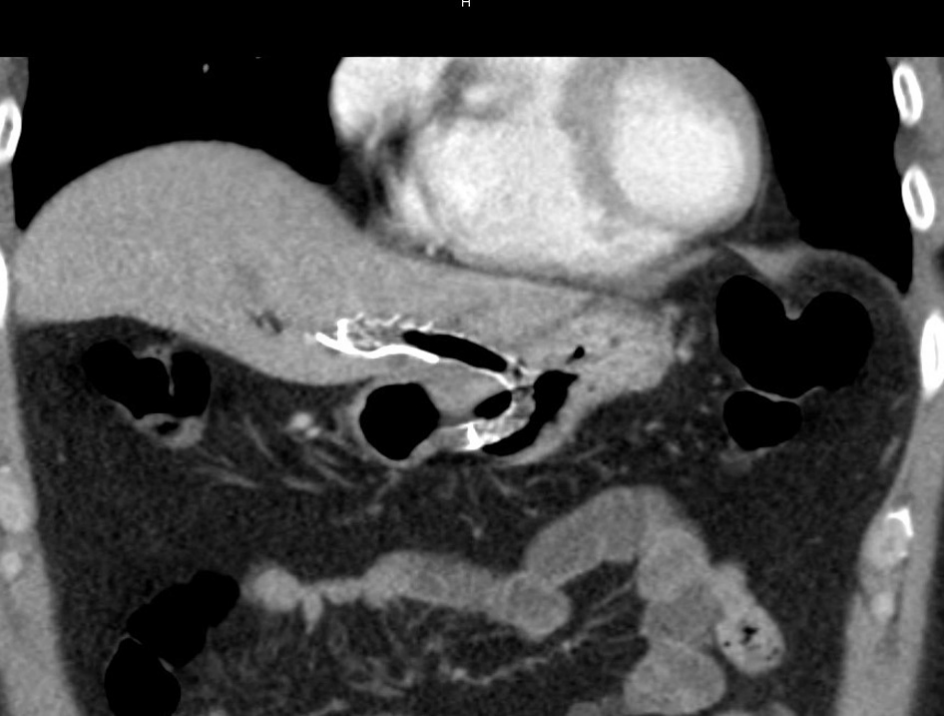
CT image of the Viabil stent across the hepaticogastrostomy.
